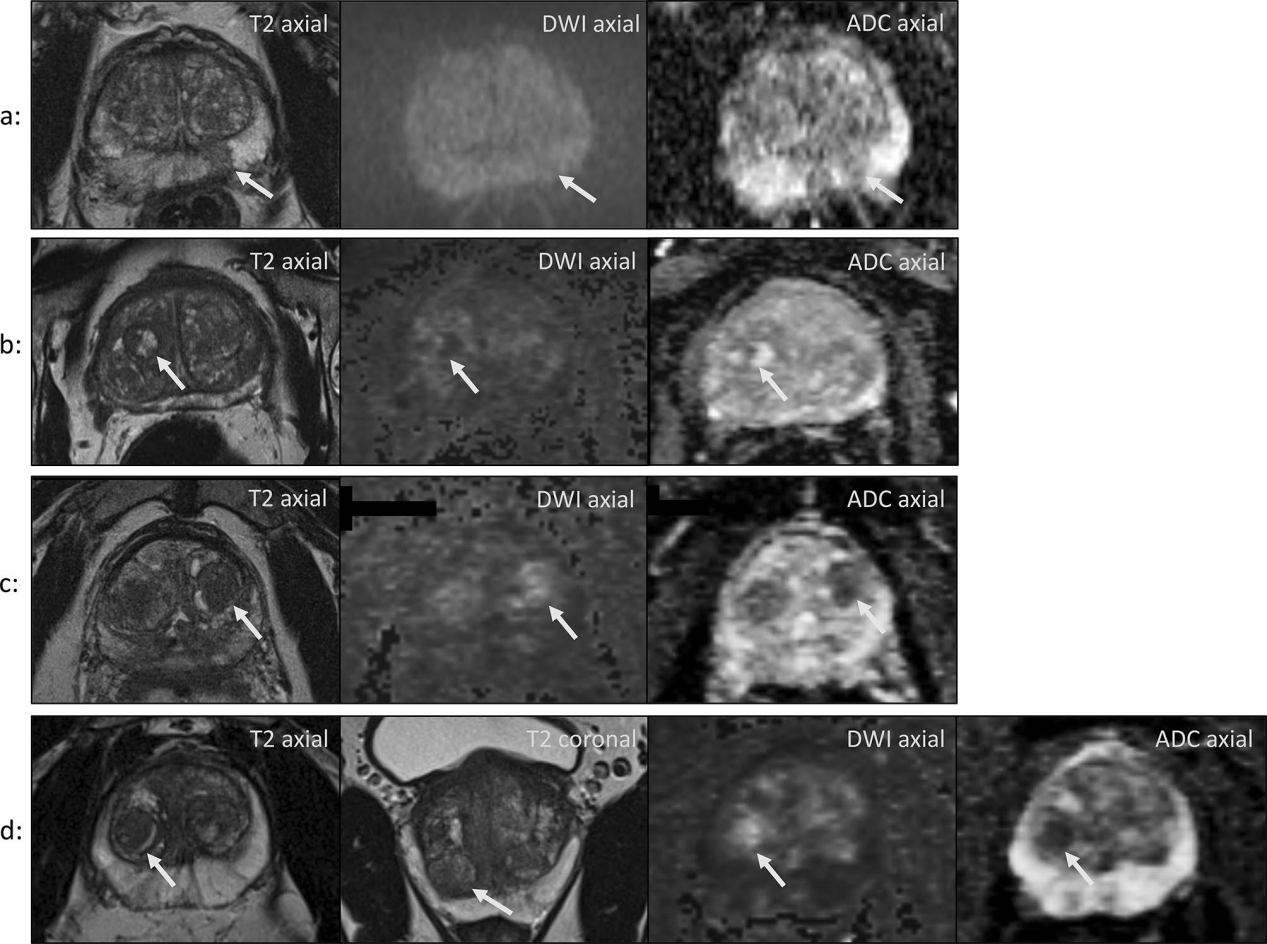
(a) Oval-shaped lesion in the left PZ with DWI isointensity and mild indistinct ADC hypointensity. It was scored a 2 according to PI-RADS v2.0 and a 3 according to PI-RADS v2.1. TB of this lesion revealed no cancer. Patient age: 68 years. (b) Fully encapsulated and heterogeneous nodule in the right TZ with a diameter of 1.5 cm. It shows heterogeneous signal on high b-value DWI and on ADC images. It was scored into category 2 according to PI-RADS v2.0 and into category 1 according to PI-RADS v2.1 and showed no cancer upon systematic biopsy. Patient age: 56 years. (c) Incompletely encapsulated nodule in the left TZ with marked DWI hyperintensity and marked ADC hypointensity. Patient age: 59 years. (d) Lesion in the right apical TZ with the same characteristics as in c. Patient age: 52 years. The new DWI upgrading rule was applied to lesions in c and d. In both cases systematic biopsy showed no cancer.
